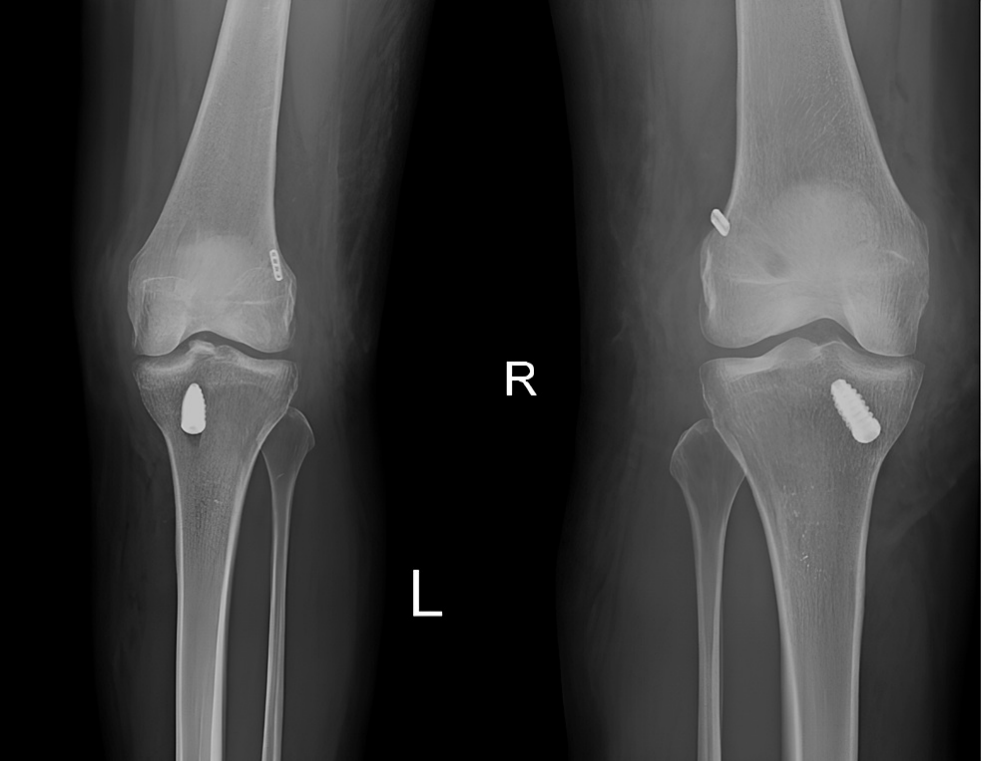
Post-operative X-ray bilateral knee AP view AP: Anterior–posterior
